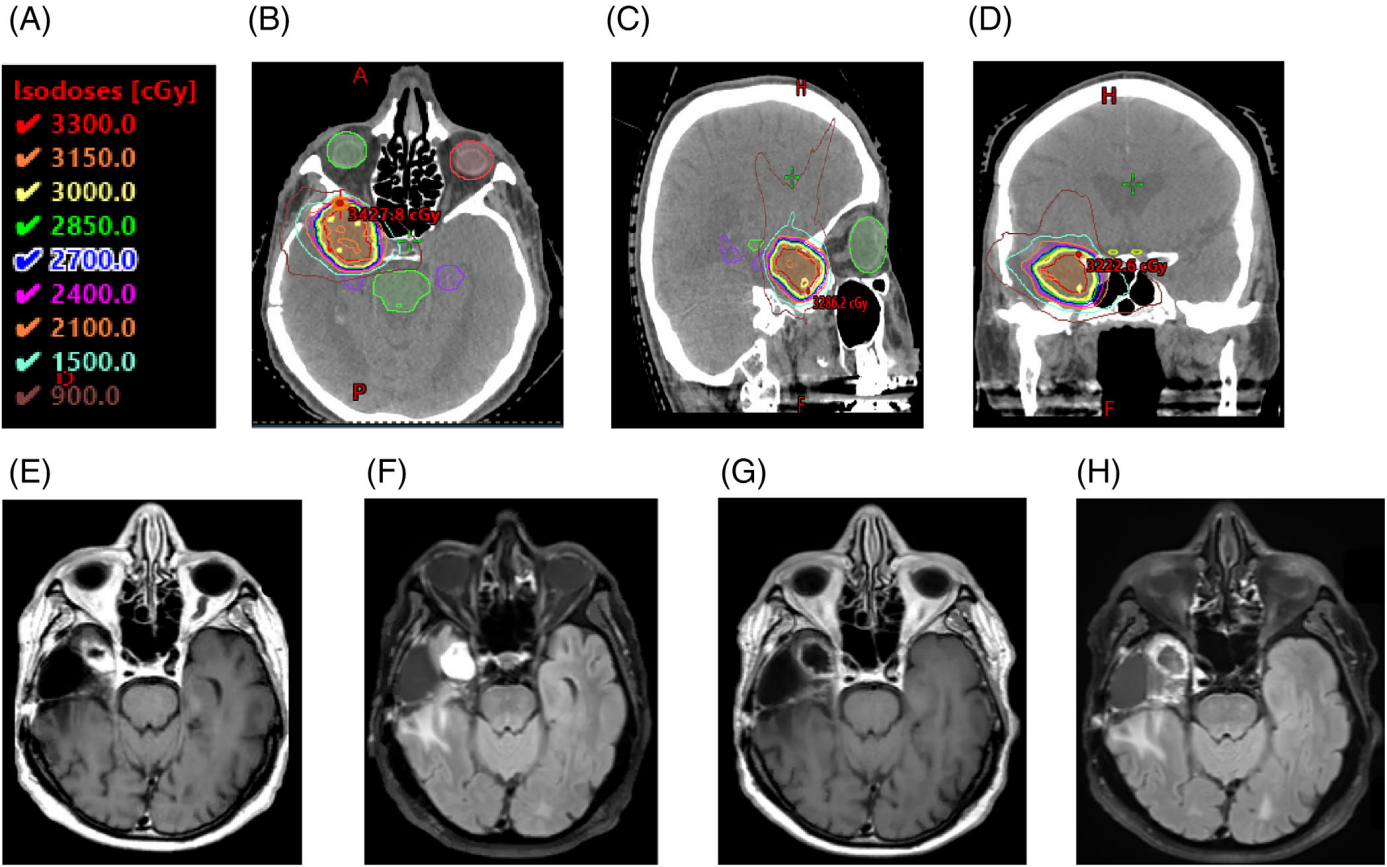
Partial Response in Patient Receiving ICI + SBRT. 83‐year‐old man with right temporal WHO grade IV glioblastoma status‐post resection and adjuvant concurrent radiotherapy and temozolomide who received 30 Gy in five fractions concurrently with 15 cycles of ICI as his third line treatment. Radiotherapy isodose line key shown in (A). Representative (B) axial (C) sagittal and (D) coronal images of his RT plan. Pretreatment MRI (E) axial T1 and (F) axial T2 FLAIR showing 2 × 2.1 cm nodular enhancing mass along anterior/medial margin of the resection cavity. MRI 4 months post‐SBRT (G) axial T1 and (H) axial T2 FLAIR showing overall decrease in size and nodular enhancing component of the lesion.
